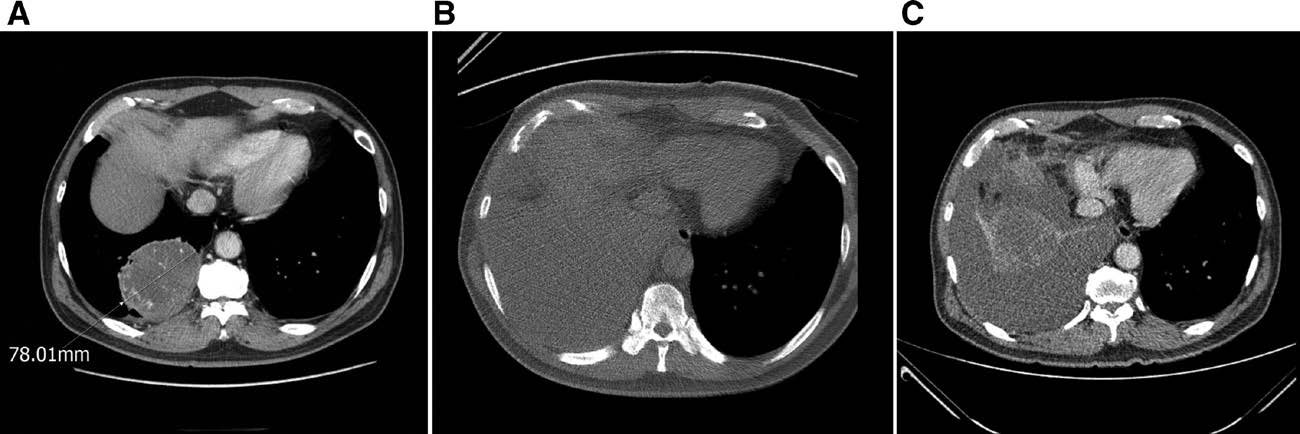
(A) CT scan of the thorax revealed a right lower lung mass. (B). There were large amounts of pleural effusion and target mass hard to distinguish on CT performed during a lung biopsy attempt. (C) There was a large right pleural effusion with compressive atelectasis and mass-like opacity at follow-up enhanced chest CT. CT = computed tomography.
